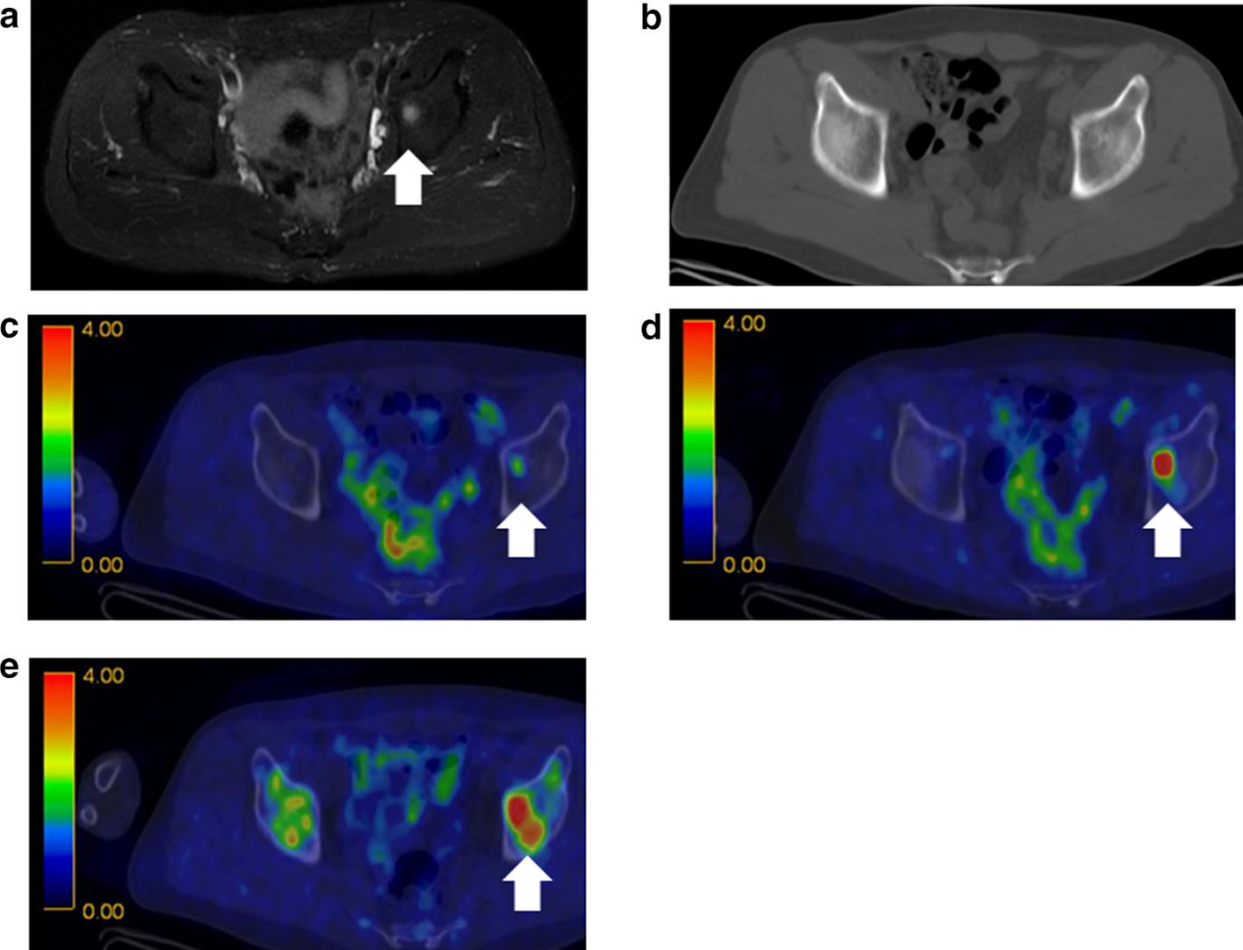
a CT and b MRI fusion images of c
